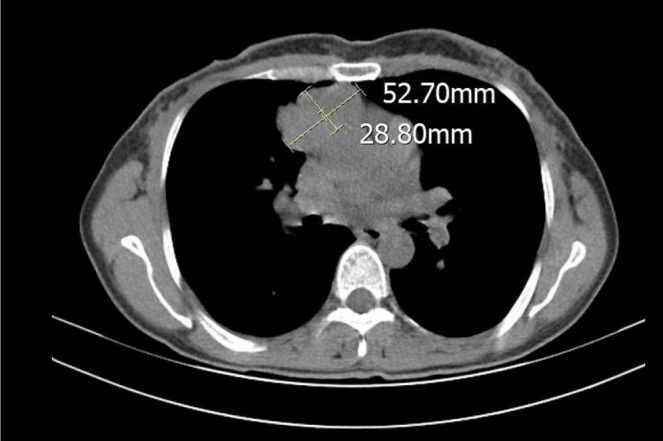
Contrast‐enhanced chest computed tomography (CT) (axial view) demonstrating a heterogeneous anterior mediastinal mass measuring 52 × 28 mm.
